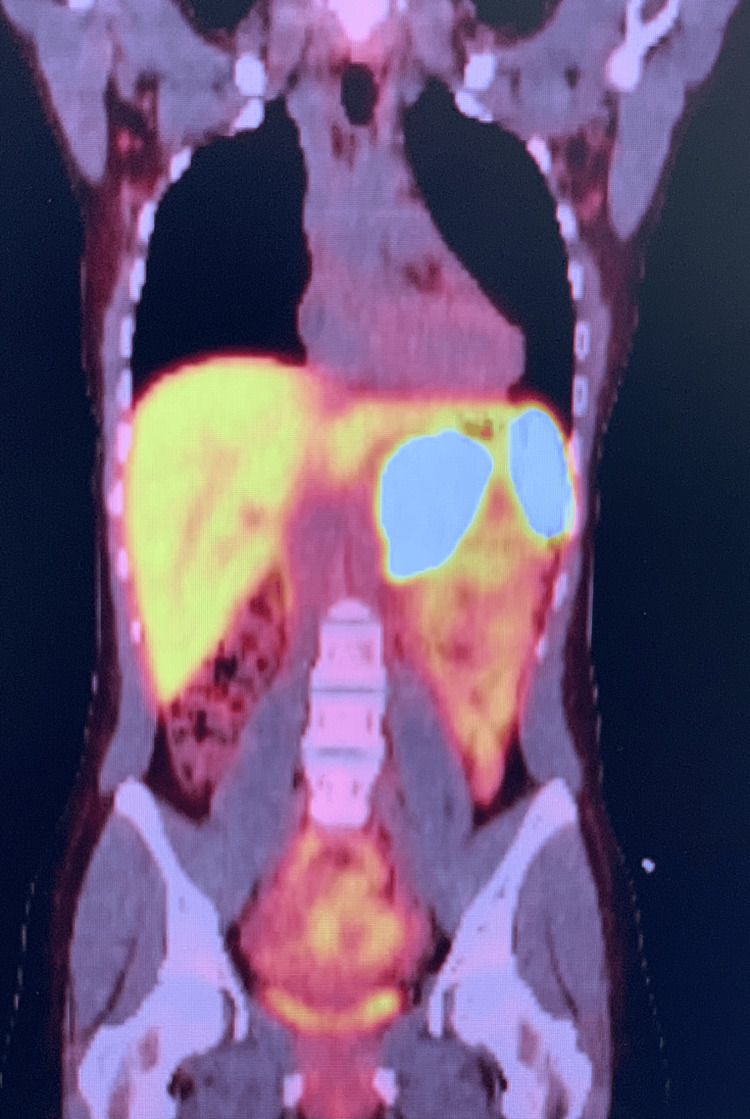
Nuclear medicine dotatate positron emission tomography-computed tomography
